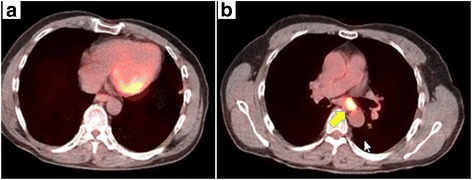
Positron emission tomography. a, b FDG uptake was detected in a subcarinal lymph node and not in a nodule in the lingular segment. The arrow indicates the lymph node with FDG accumulation
